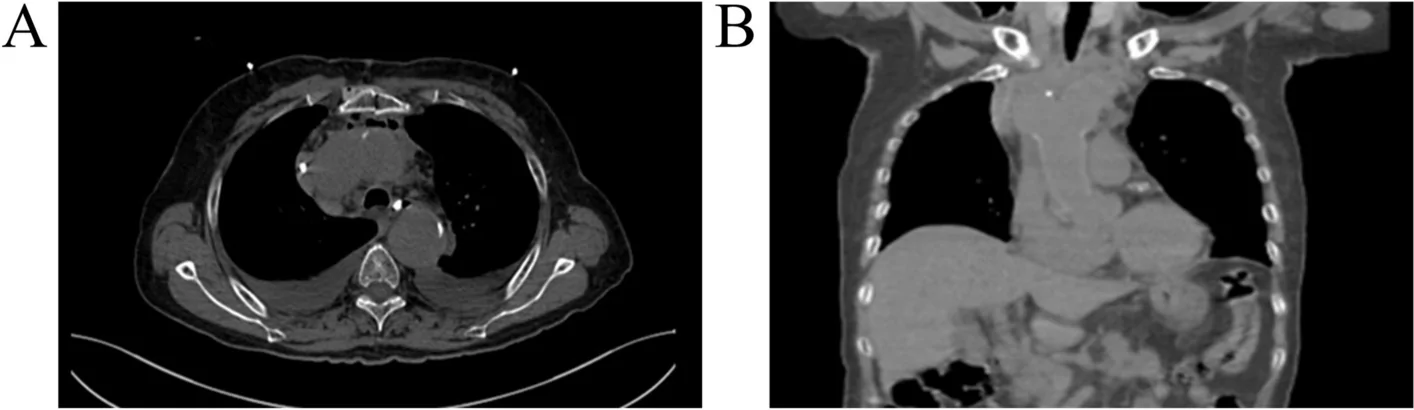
A, B Non-contrast chest CT on postoperative day 5 shows no remarkable abnormalities
